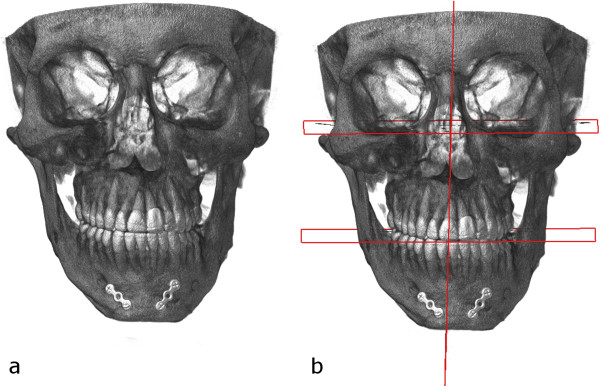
Postoperative CT scan (a) with planes defined (b).
